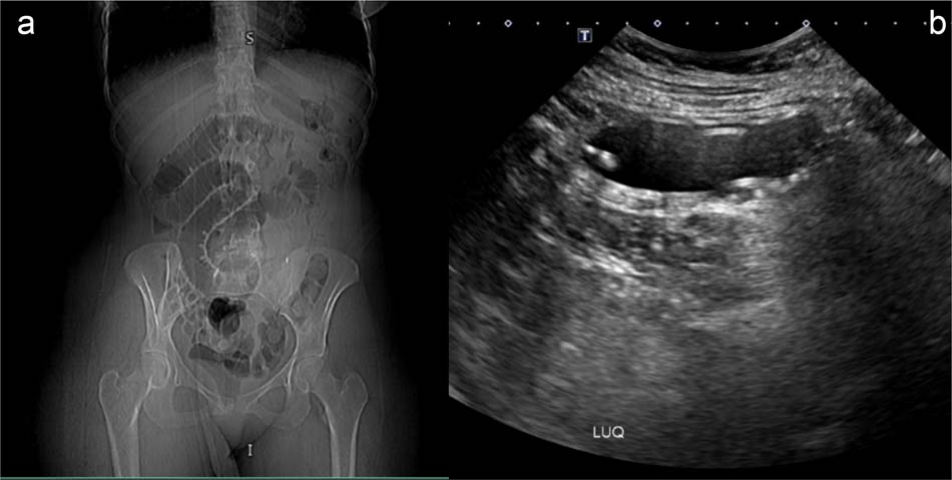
(a) Plan X-ray abdomen showing dilated jejunal loops. (b) Abdominal US showing small bowel containing IGB.
